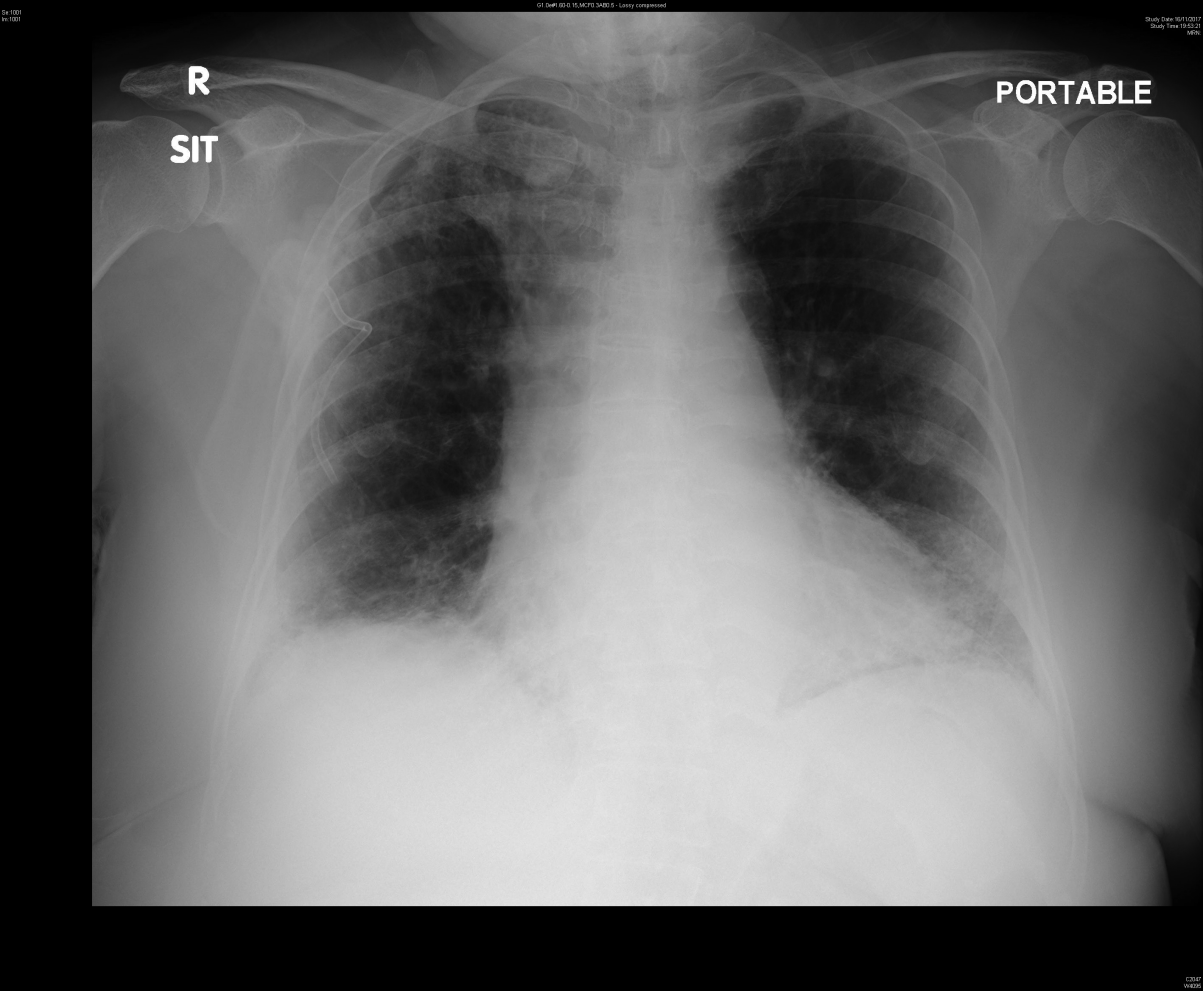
Chest radiograph after oxytetracycline pleurodesis with Rocket® Pleural Vent™ removed, showing full lung re‐expansion.
